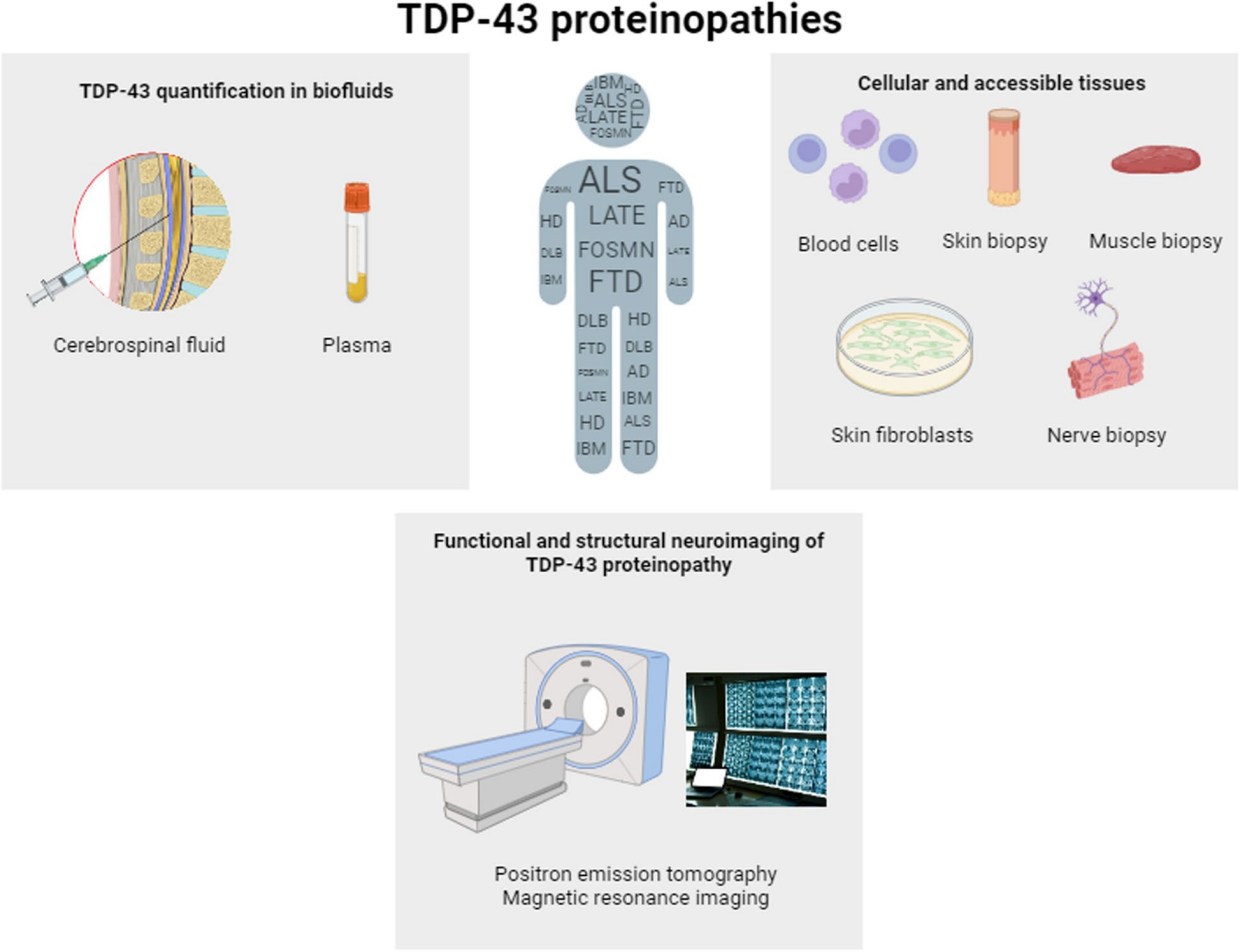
An overview of the different TDP-43 detection approaches considered for diagnostic use, including quantification of free TDP-43 in biofluids (i.e., plasma/serum and CSF) and extracellular vesicles, structural and functional neuroimaging with magnetic resonance imaging and positron emission tomography, and detection of TDP-43 pathology in cells and tissues outside the CNS (blood cells, skin fibroblasts), and other tissues, such as muscle and nerve
